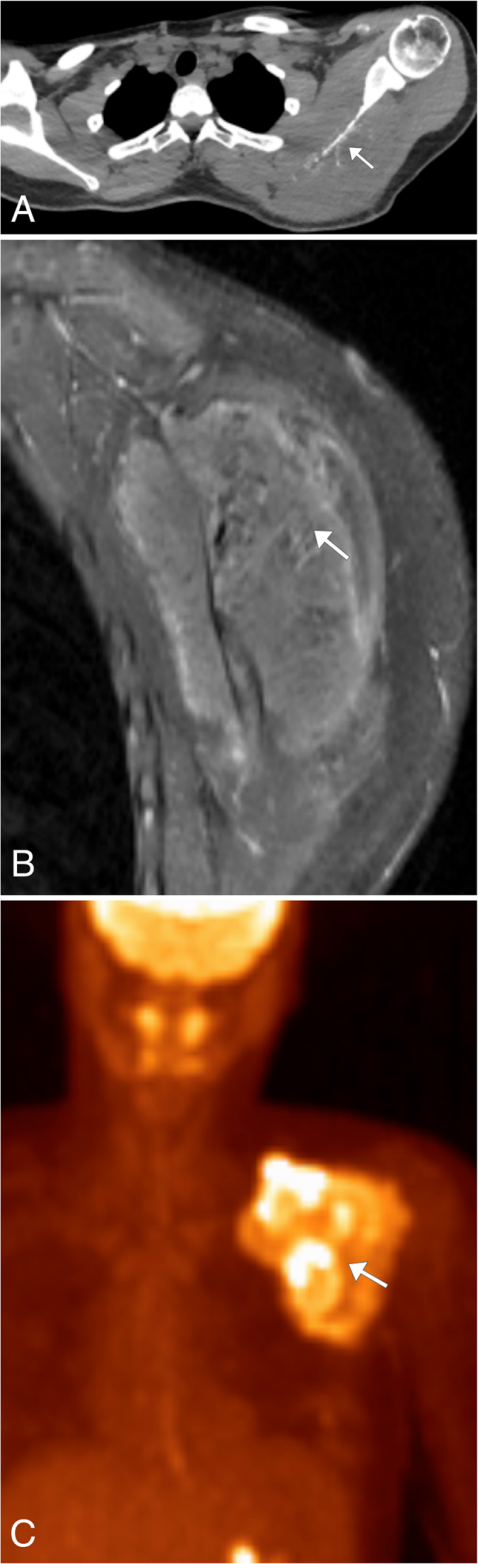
Ewing sarcoma. a Axial noncontrast chest CT demonstrates permeative destructive changes of the left scapula (arrow) with the large soft tissue component of the mass not well seen. b Sagittal T1WI MR image post contrast allows for visualization of the associated enhancing mass (arrow) which involves the surrounding left scapular soft tissues and musculature. c Coronal FDG-PET/CT image demonstrating the hypermetabolic nature of the lesion associated with the left scapula (arrow)
